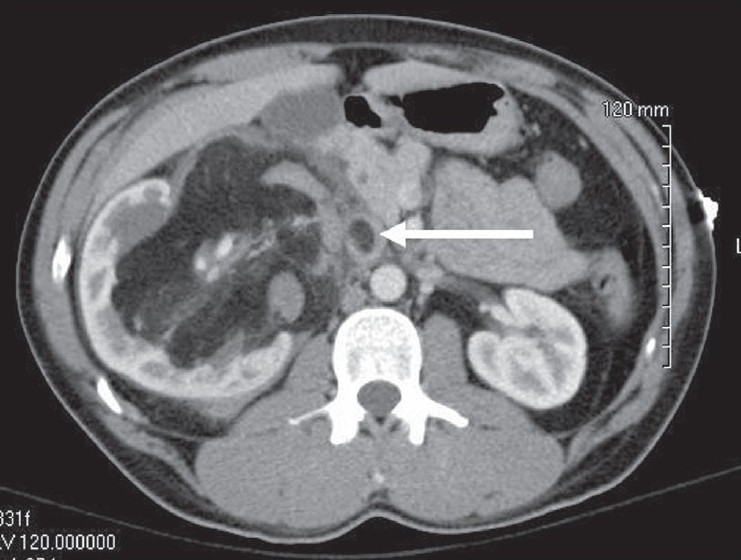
A contrast-enhanced computed tomography scan shows a mass within the right kidney and evidence of tumor thrombus within the inferior vena cava
